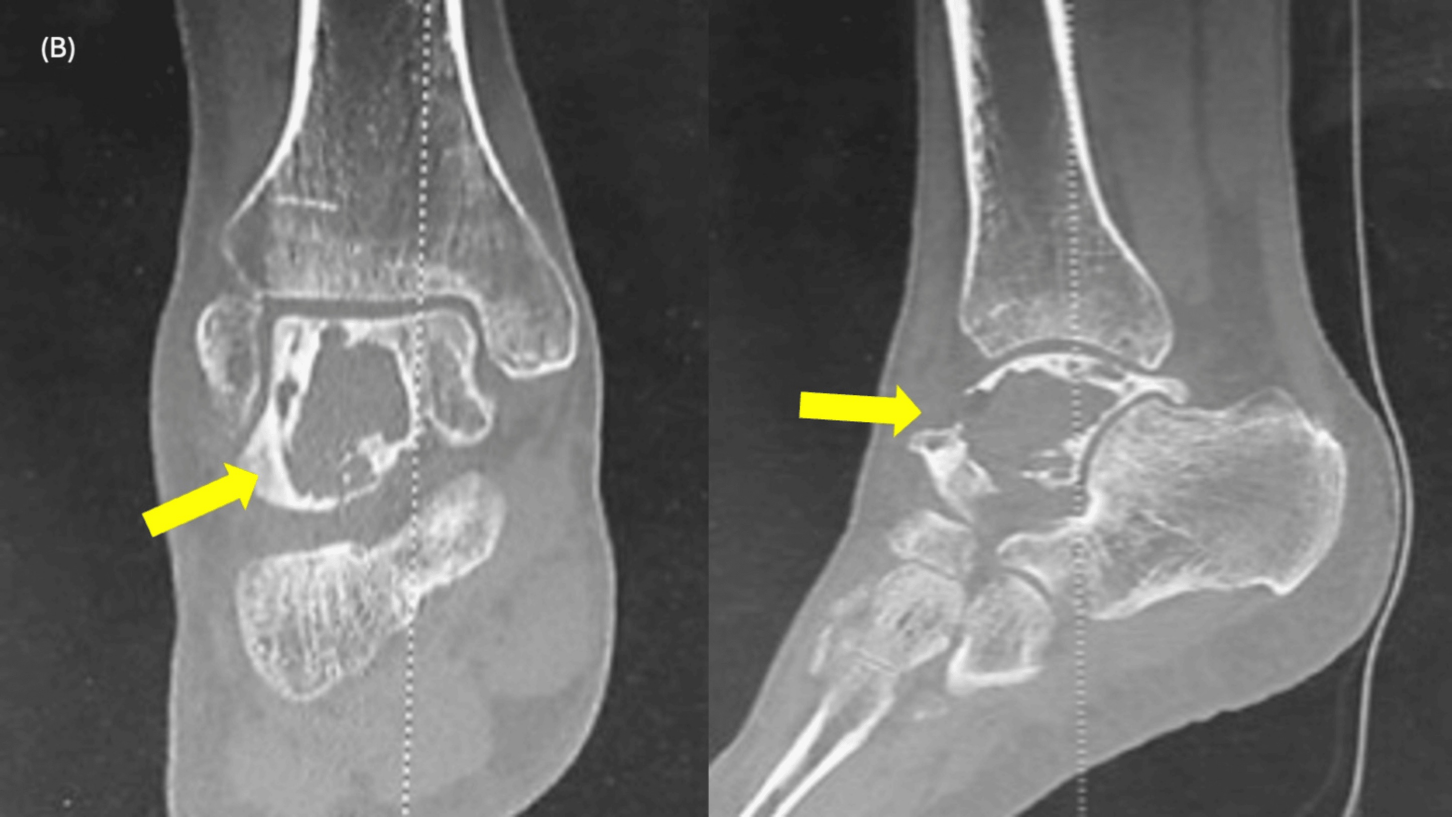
CT showing osteolytic lesion in the talus (yellow arrows) CT: computed tomography
